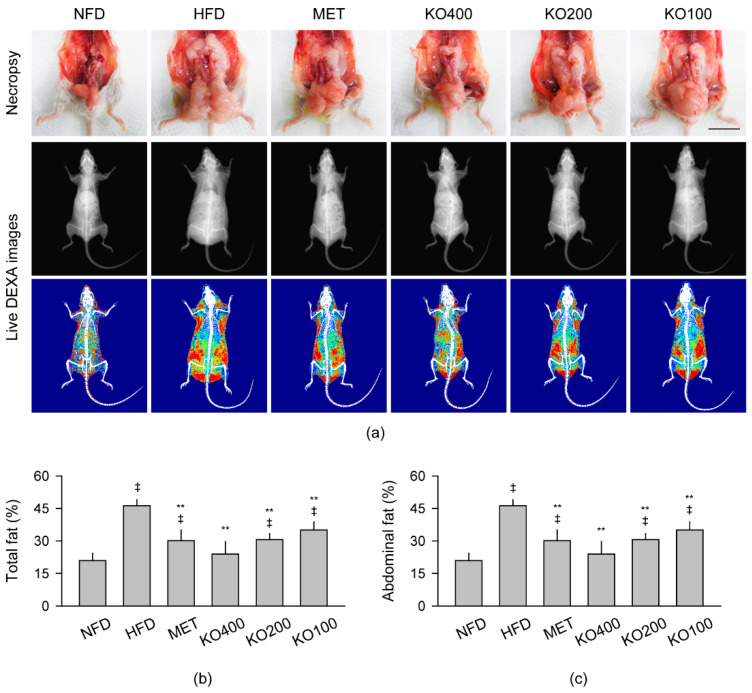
Body fat deposition: (a) Representative images of body fat deposition in necropsy and live dual-energy X-ray absorptiometry (DEXA). In the live DEXA images, red, yellow, and blue indicate high-, intermediate-, and low-density fats, respectively. Scale bars = 2 cm. (b,c) Fat densities in total body and the abdominal region. Values are expressed as the mean ± SD. ^‡^
